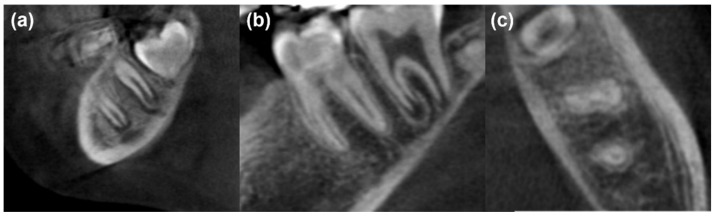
Cone-beam computed tomography (CBCT) analysis for some teeth after 12 months. (a) Sagittal view of incomplete apical closure in pulpotomy treatment using PRF; (b) sagittal view of complete apical closure in pulpotomy treatment using PRF; (c) axial view of complete apical closure in pulpotomy treatment using PRF.
